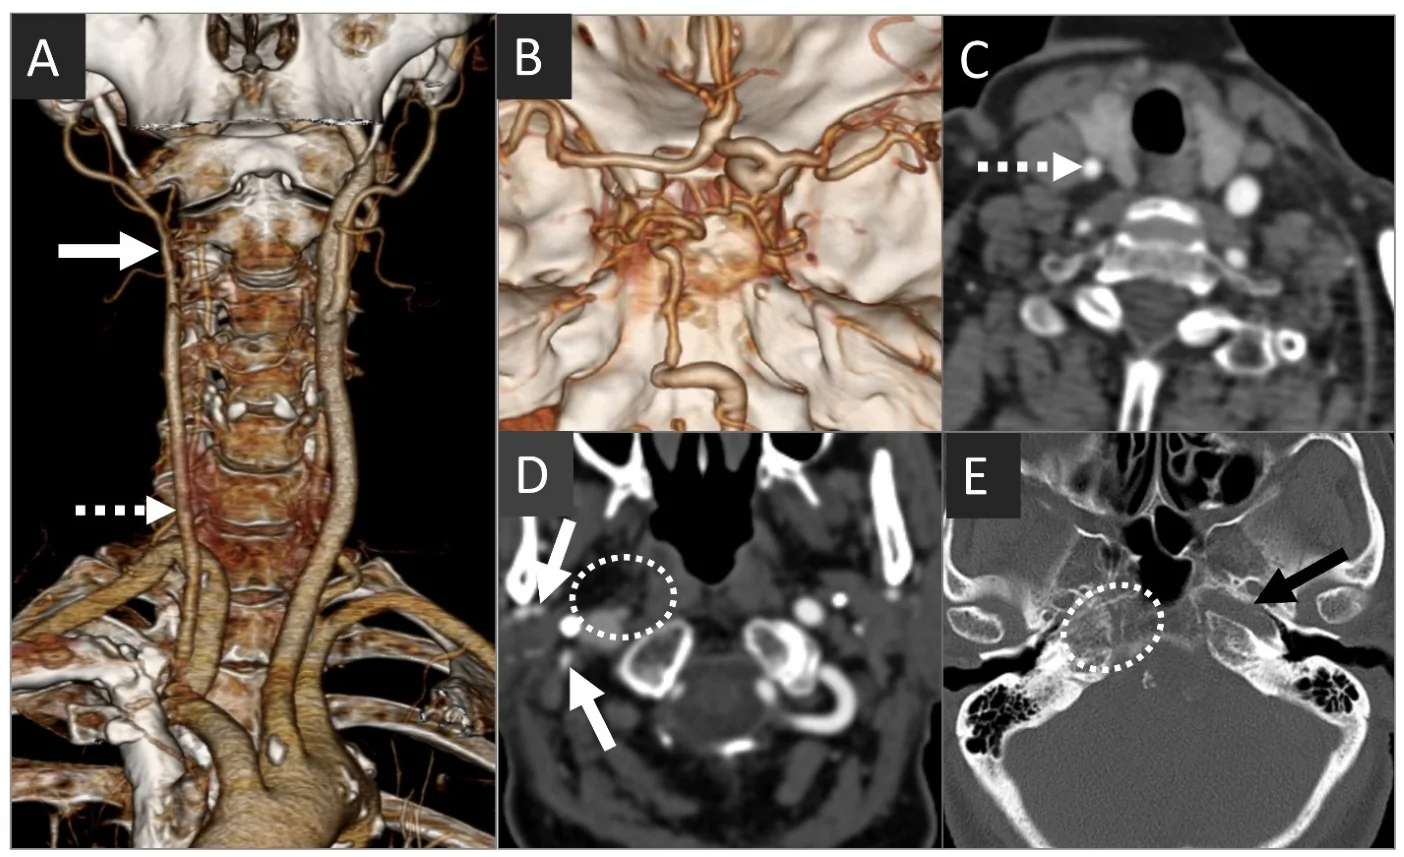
Right ICA agenesis. Volume-rendered (A,B) and axial (C–E) CTA images demonstrate an asymmetrically hypoplastic right CCA (dashed arrows) that continues directly as the ECA (white arrows), with an absent right ICA (white circle in (D)). The right bony carotid canal is absent (white circle in (E)), while the left canal (black arrow) is normal. The right ACA and MCA are supplied by the left ICA via the AComA, and the posterior circulation contributes through the PComA.
